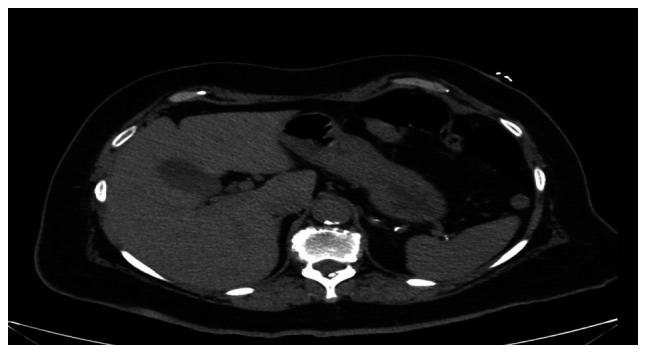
Non-contrast computed tomography scan of the abdomen demonstrating no acute intra-abdominal abnormality.
